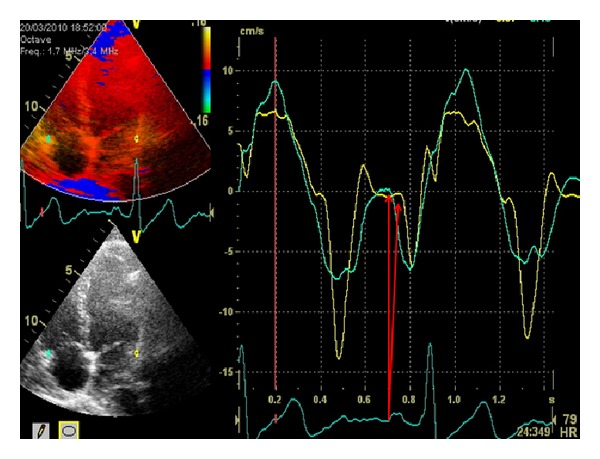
Pulsed-wave tissue Doppler early diastolic [*E*
